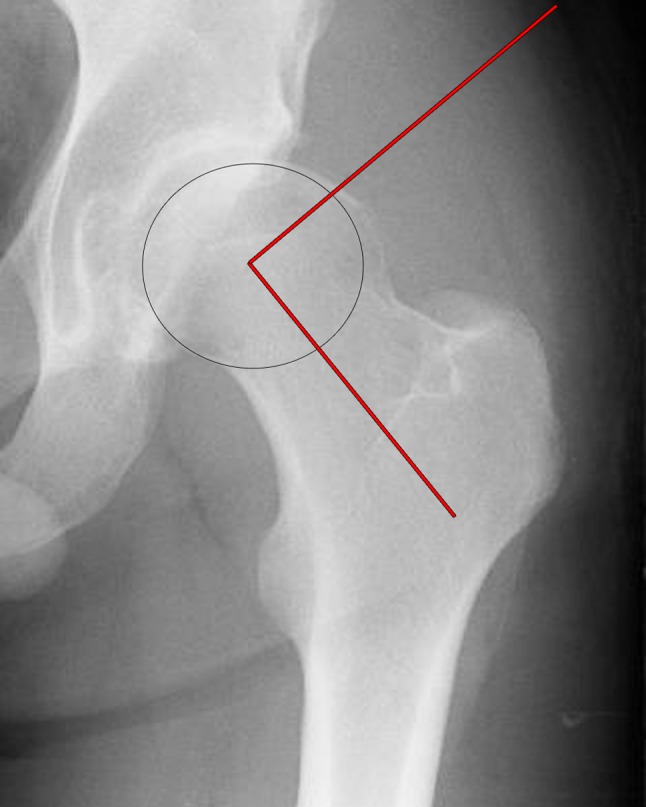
A magnified anterior posterior radiograph view taken from a pelvis radiograph with an increased alpha angle. The alpha angle subtended between a line from the midline of the femur to the center of the femoral head and a line from the center of the femoral head to the point at which the femoral head deviated from a circular template overlay
